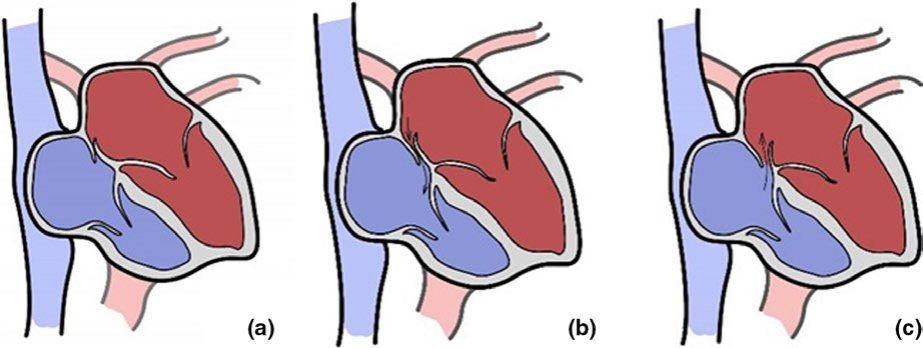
(a) Most patent foramen ovale (PFO) was a physiological closure at rest without shunts found by transesophageal echocardiography (TEE). (b) A few PFO was incomplete closure at rest and could be found left‐to‐right shunts by TEE. (c), By doing a Valsalva maneuver, the development of a right‐to‐left atrial pressure gradient results in right‐to‐left shunts, and the increased pressure of right atrial could make the PFO width lager
